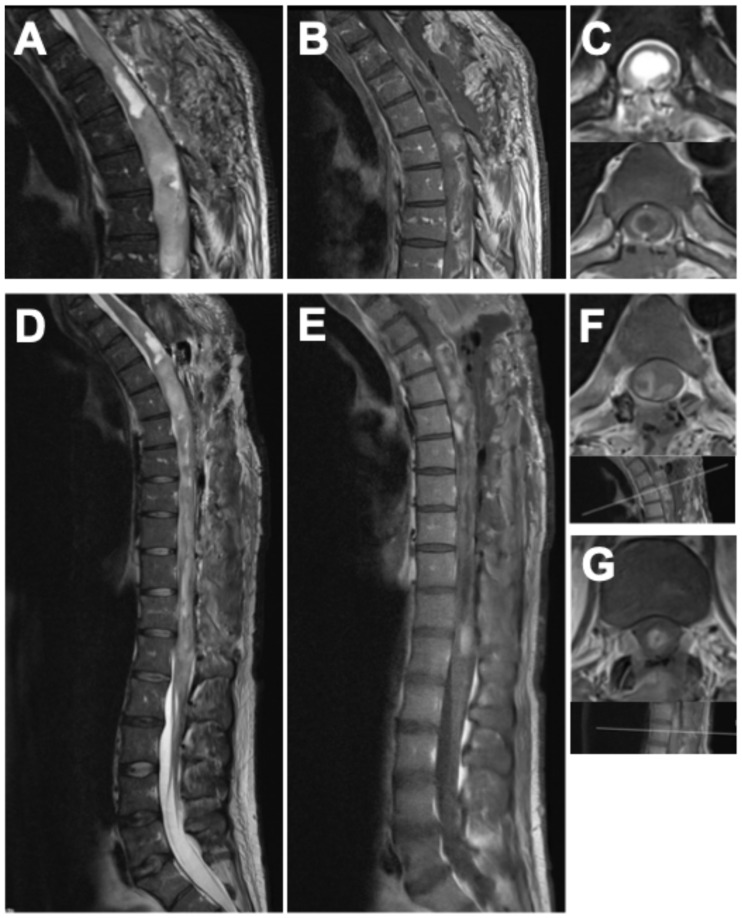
Postoperative MRI of the spine after the first surgery (A–C), showing the thoracic spine in (A) sagittal T2-weighted, (B) sagittal gadolinium-enhanced T1-weighted, and (C) axial T2- and gadolinium-enhanced T1-weighted sequences. Postoperative findings after the second surgery are displayed in (D–G): (D) sagittal T2-weighted, (E) sagittal gadolinium-enhanced T1-weighted, (F) axial gadolinium-enhanced T1-weighted MRI of the thoracic spine with indication of the imaging plane, and (G) axial gadolinium-enhanced T1-weighted MRI at the thoracolumbar junction with corresponding imaging plane. Following the second procedure, postoperative MRI confirmed only minimal residual tumor at the levels of T5–T7 and T12/L1, showing marked regression compared with preoperative imaging, accompanied by substantial decompression and regression of the syrinx.
